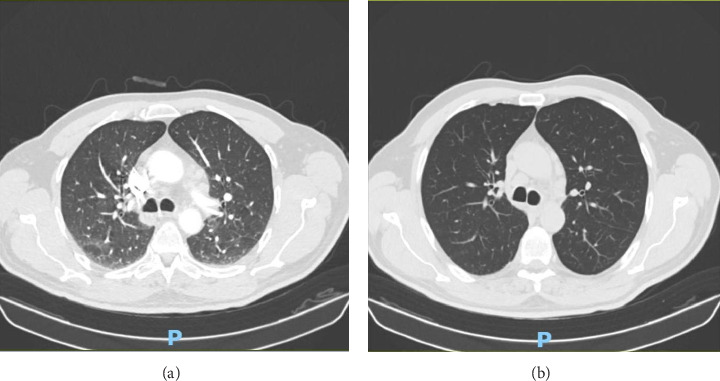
Thoracic CT scans: at baseline (a) revealing bilateral pneumonia and at 14 days (b) revealing radiological resolution.
